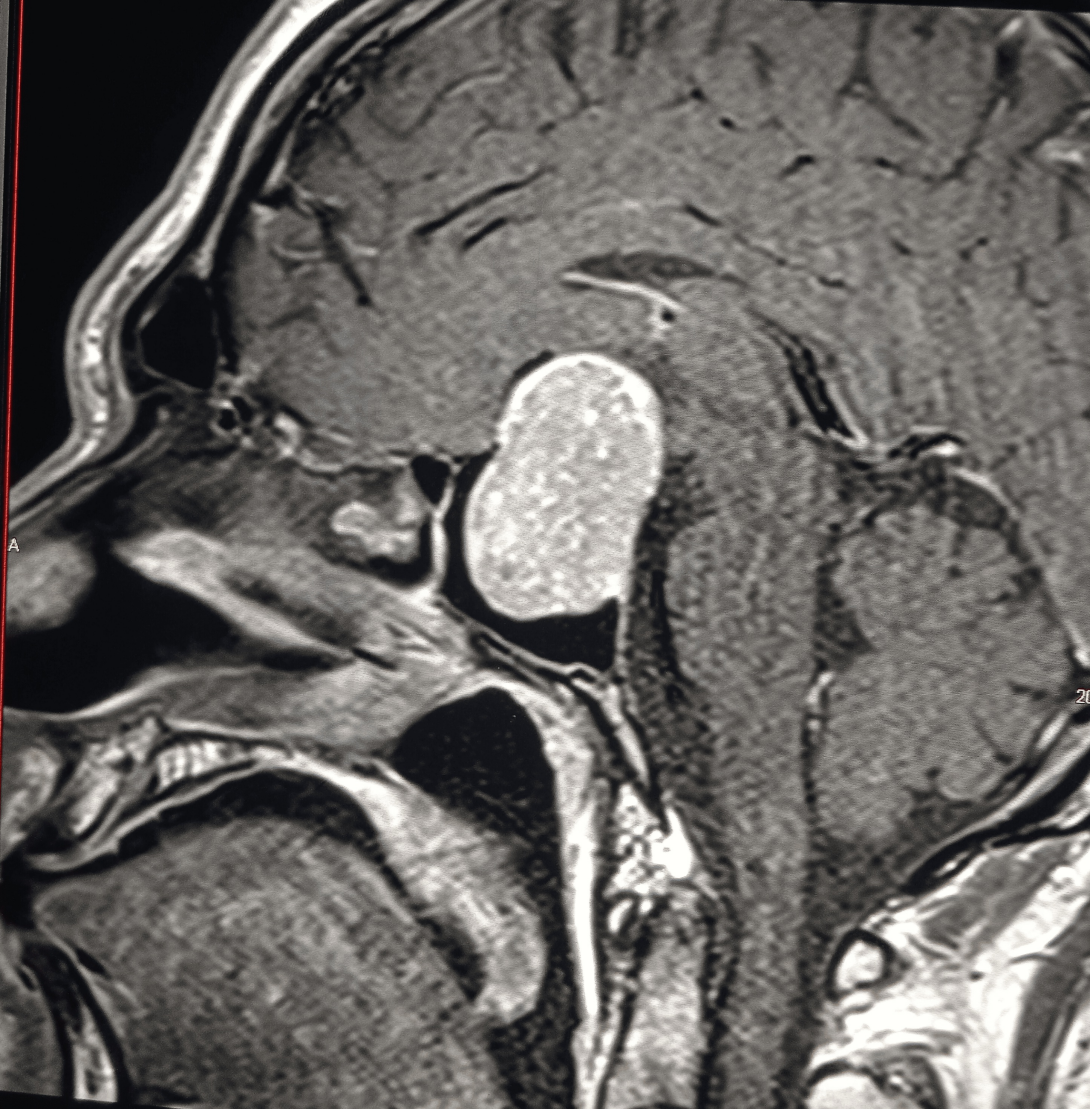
Pituitary MRI - sagittal view Before the treatment.
